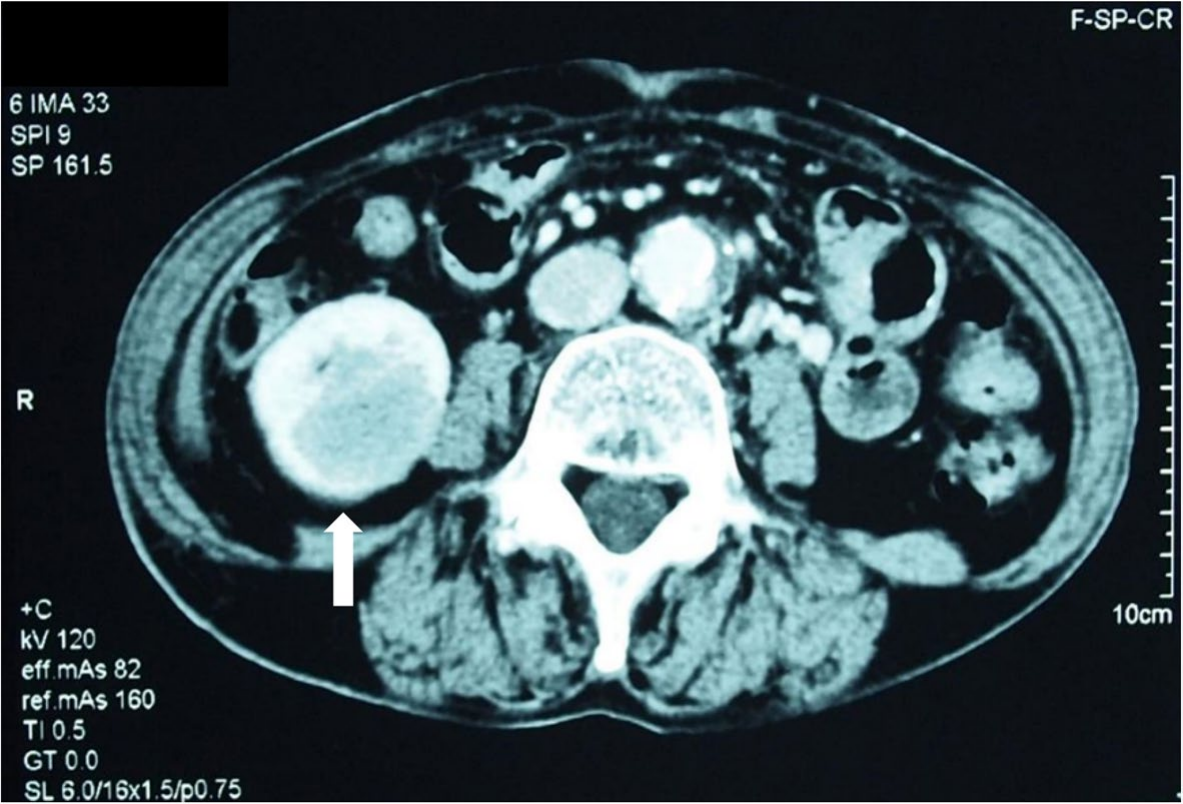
Contrast-enhanced computed tomographic scan showing a low-density right renal mass (arrow). In the inferior pole of the right kidney, a solitary and localized area of interstitial fibrosis measuring 40 × 35 × 30 mm is observed to extend from the cortex to the medulla
